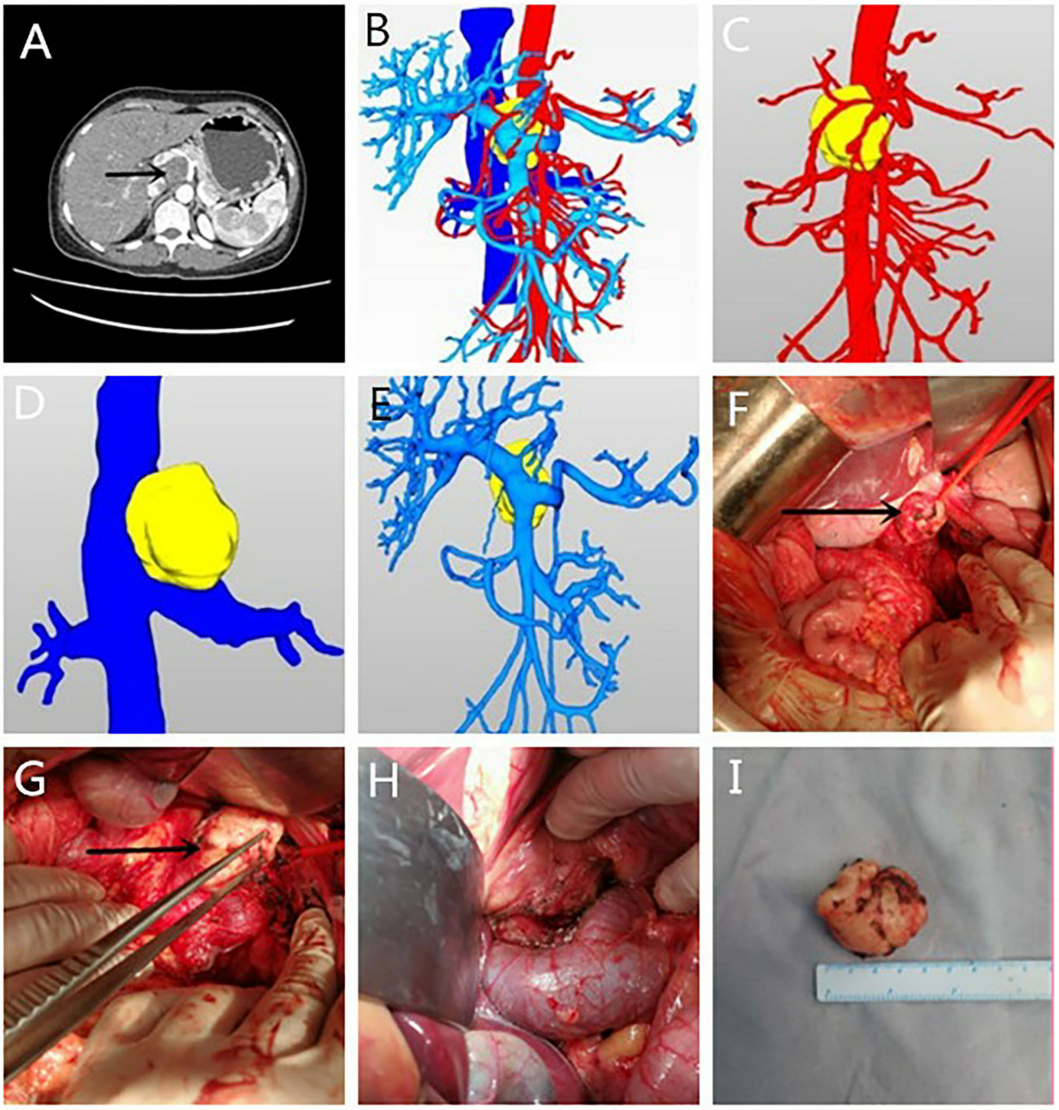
(A–I) The enhanced Computed Tomography (CT) and three-dimensional reconstruction performance and the intraoperative situation of case 3.
